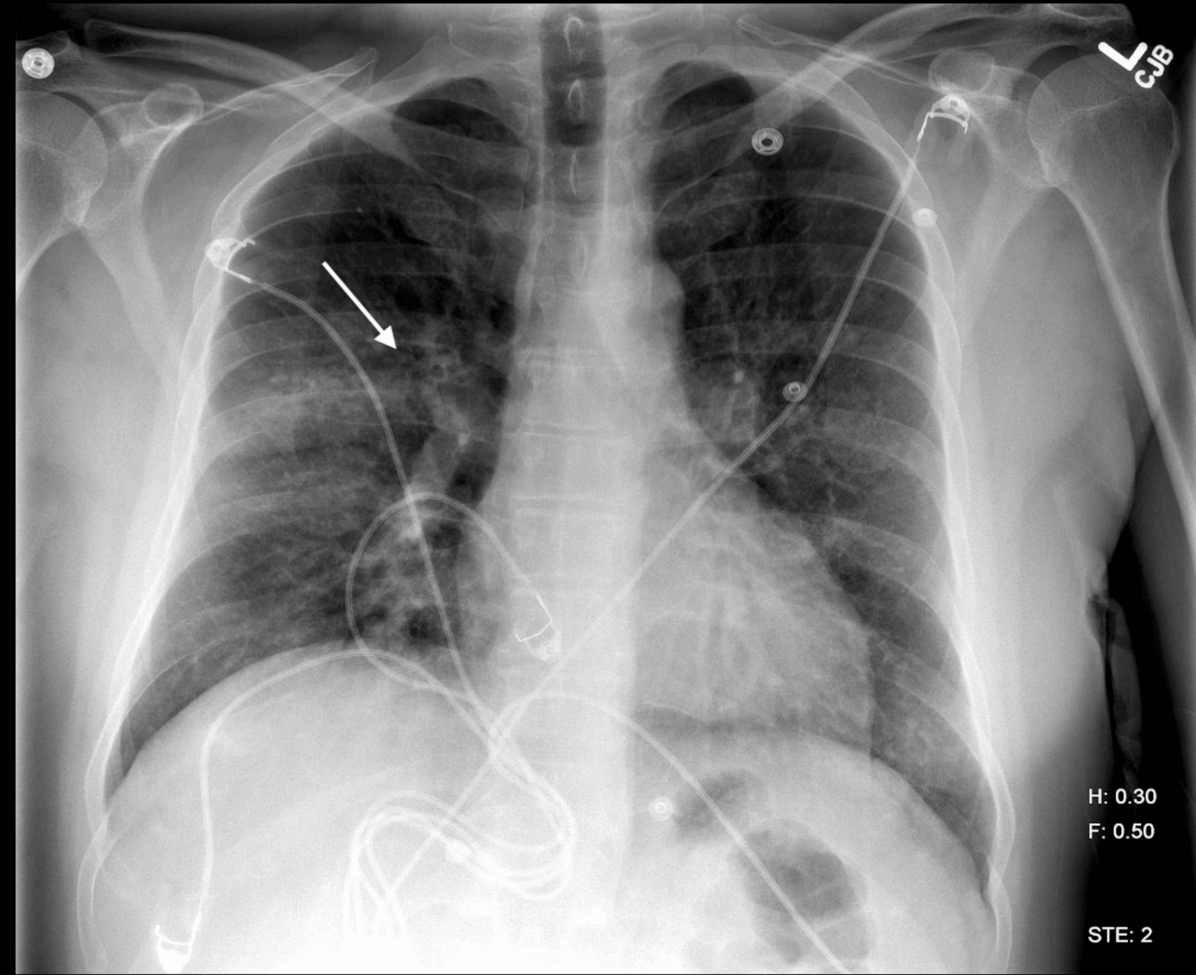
Chest radiograph demonstrates a right perihilar lower lobe infiltrate and ill-defined opacity in the left lower lobe, indicating possible infectious etiology.
